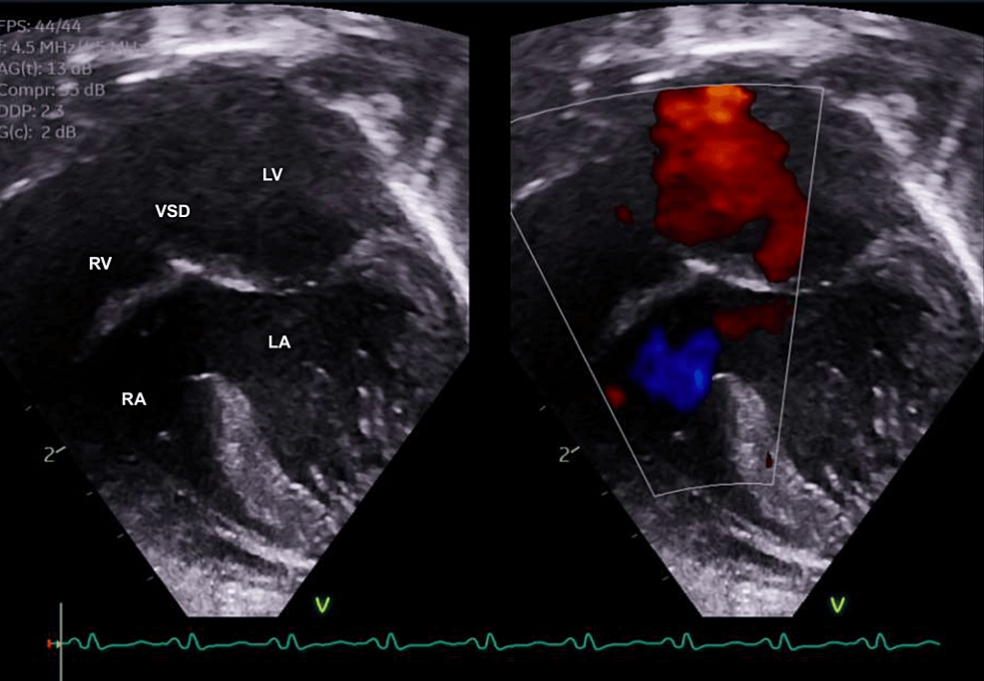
Selected video frames from the patient’s TTE at one day of age showing the patient’s hypoplastic RV paired with a plate-like atretic tricuspid valve and muscular VSD. TTE: transthoracic echocardiogram, RA: right atrium; RV: right ventricle; LA: left atrium; LV: left ventricle; VSD: ventricular septal defect
